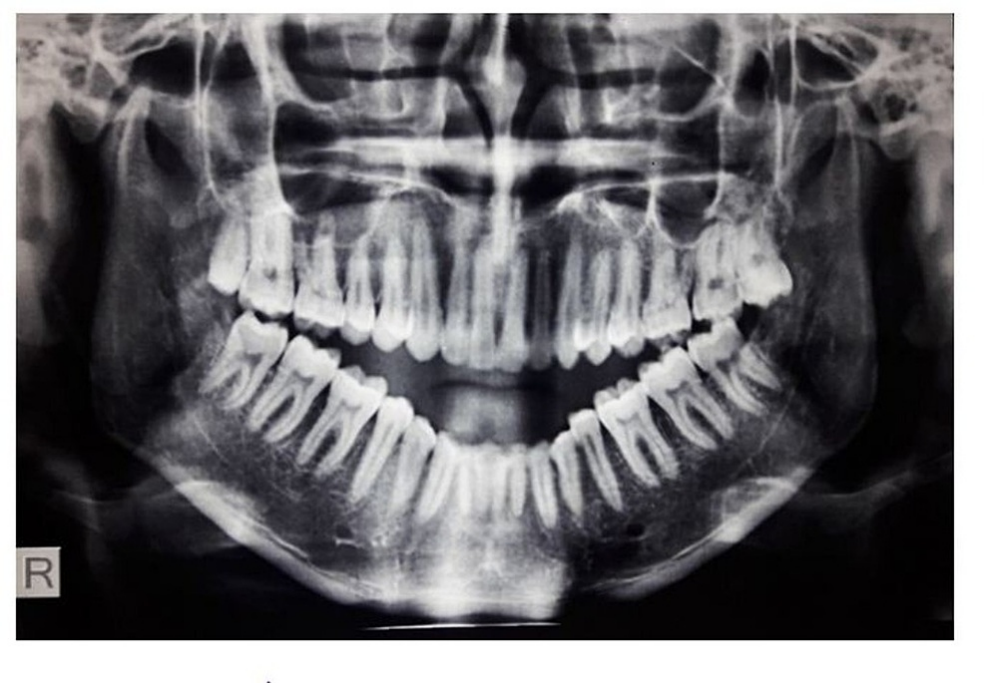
Preoperative radiograph showing bilateral anterosuperior condylar dislocation
